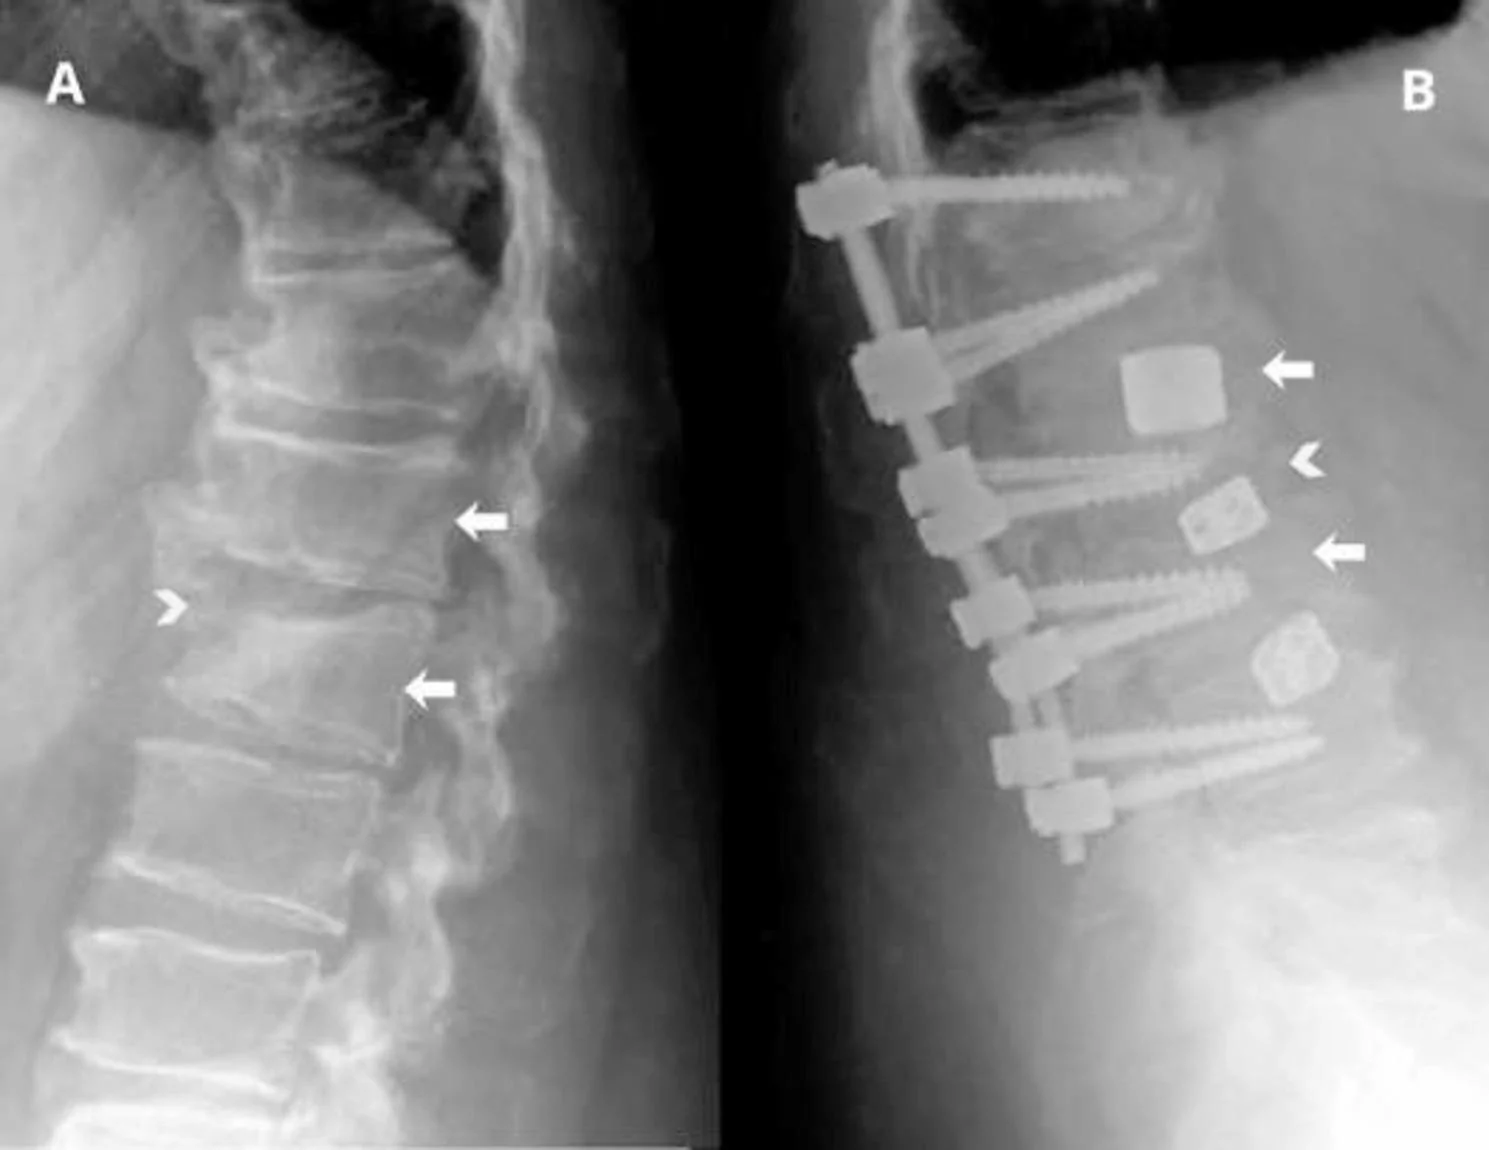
Lateral X-ray of the lumbar spine. (A) Performed at admission. (B) Postsurgical changes. Arrowhead indicates disc space. Long arrows indicate vertebrae L1 and L2
